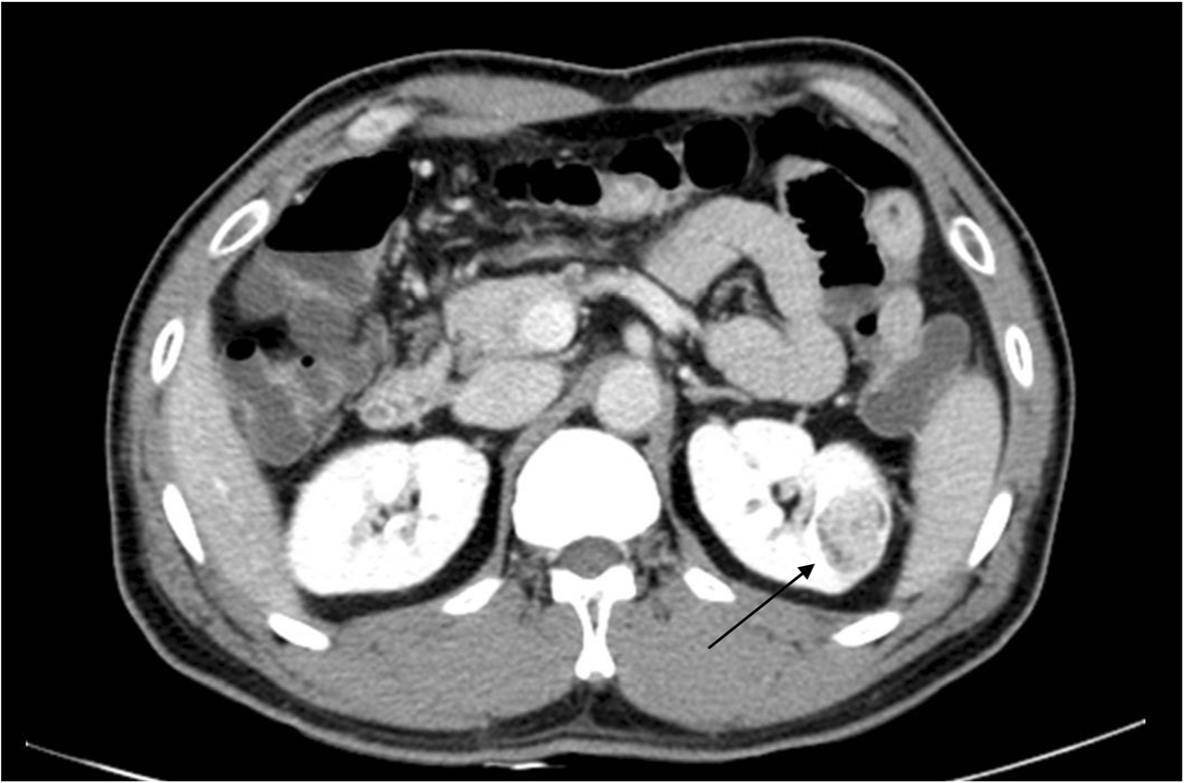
CT scan of renal cell carcinoma (Arrow)
